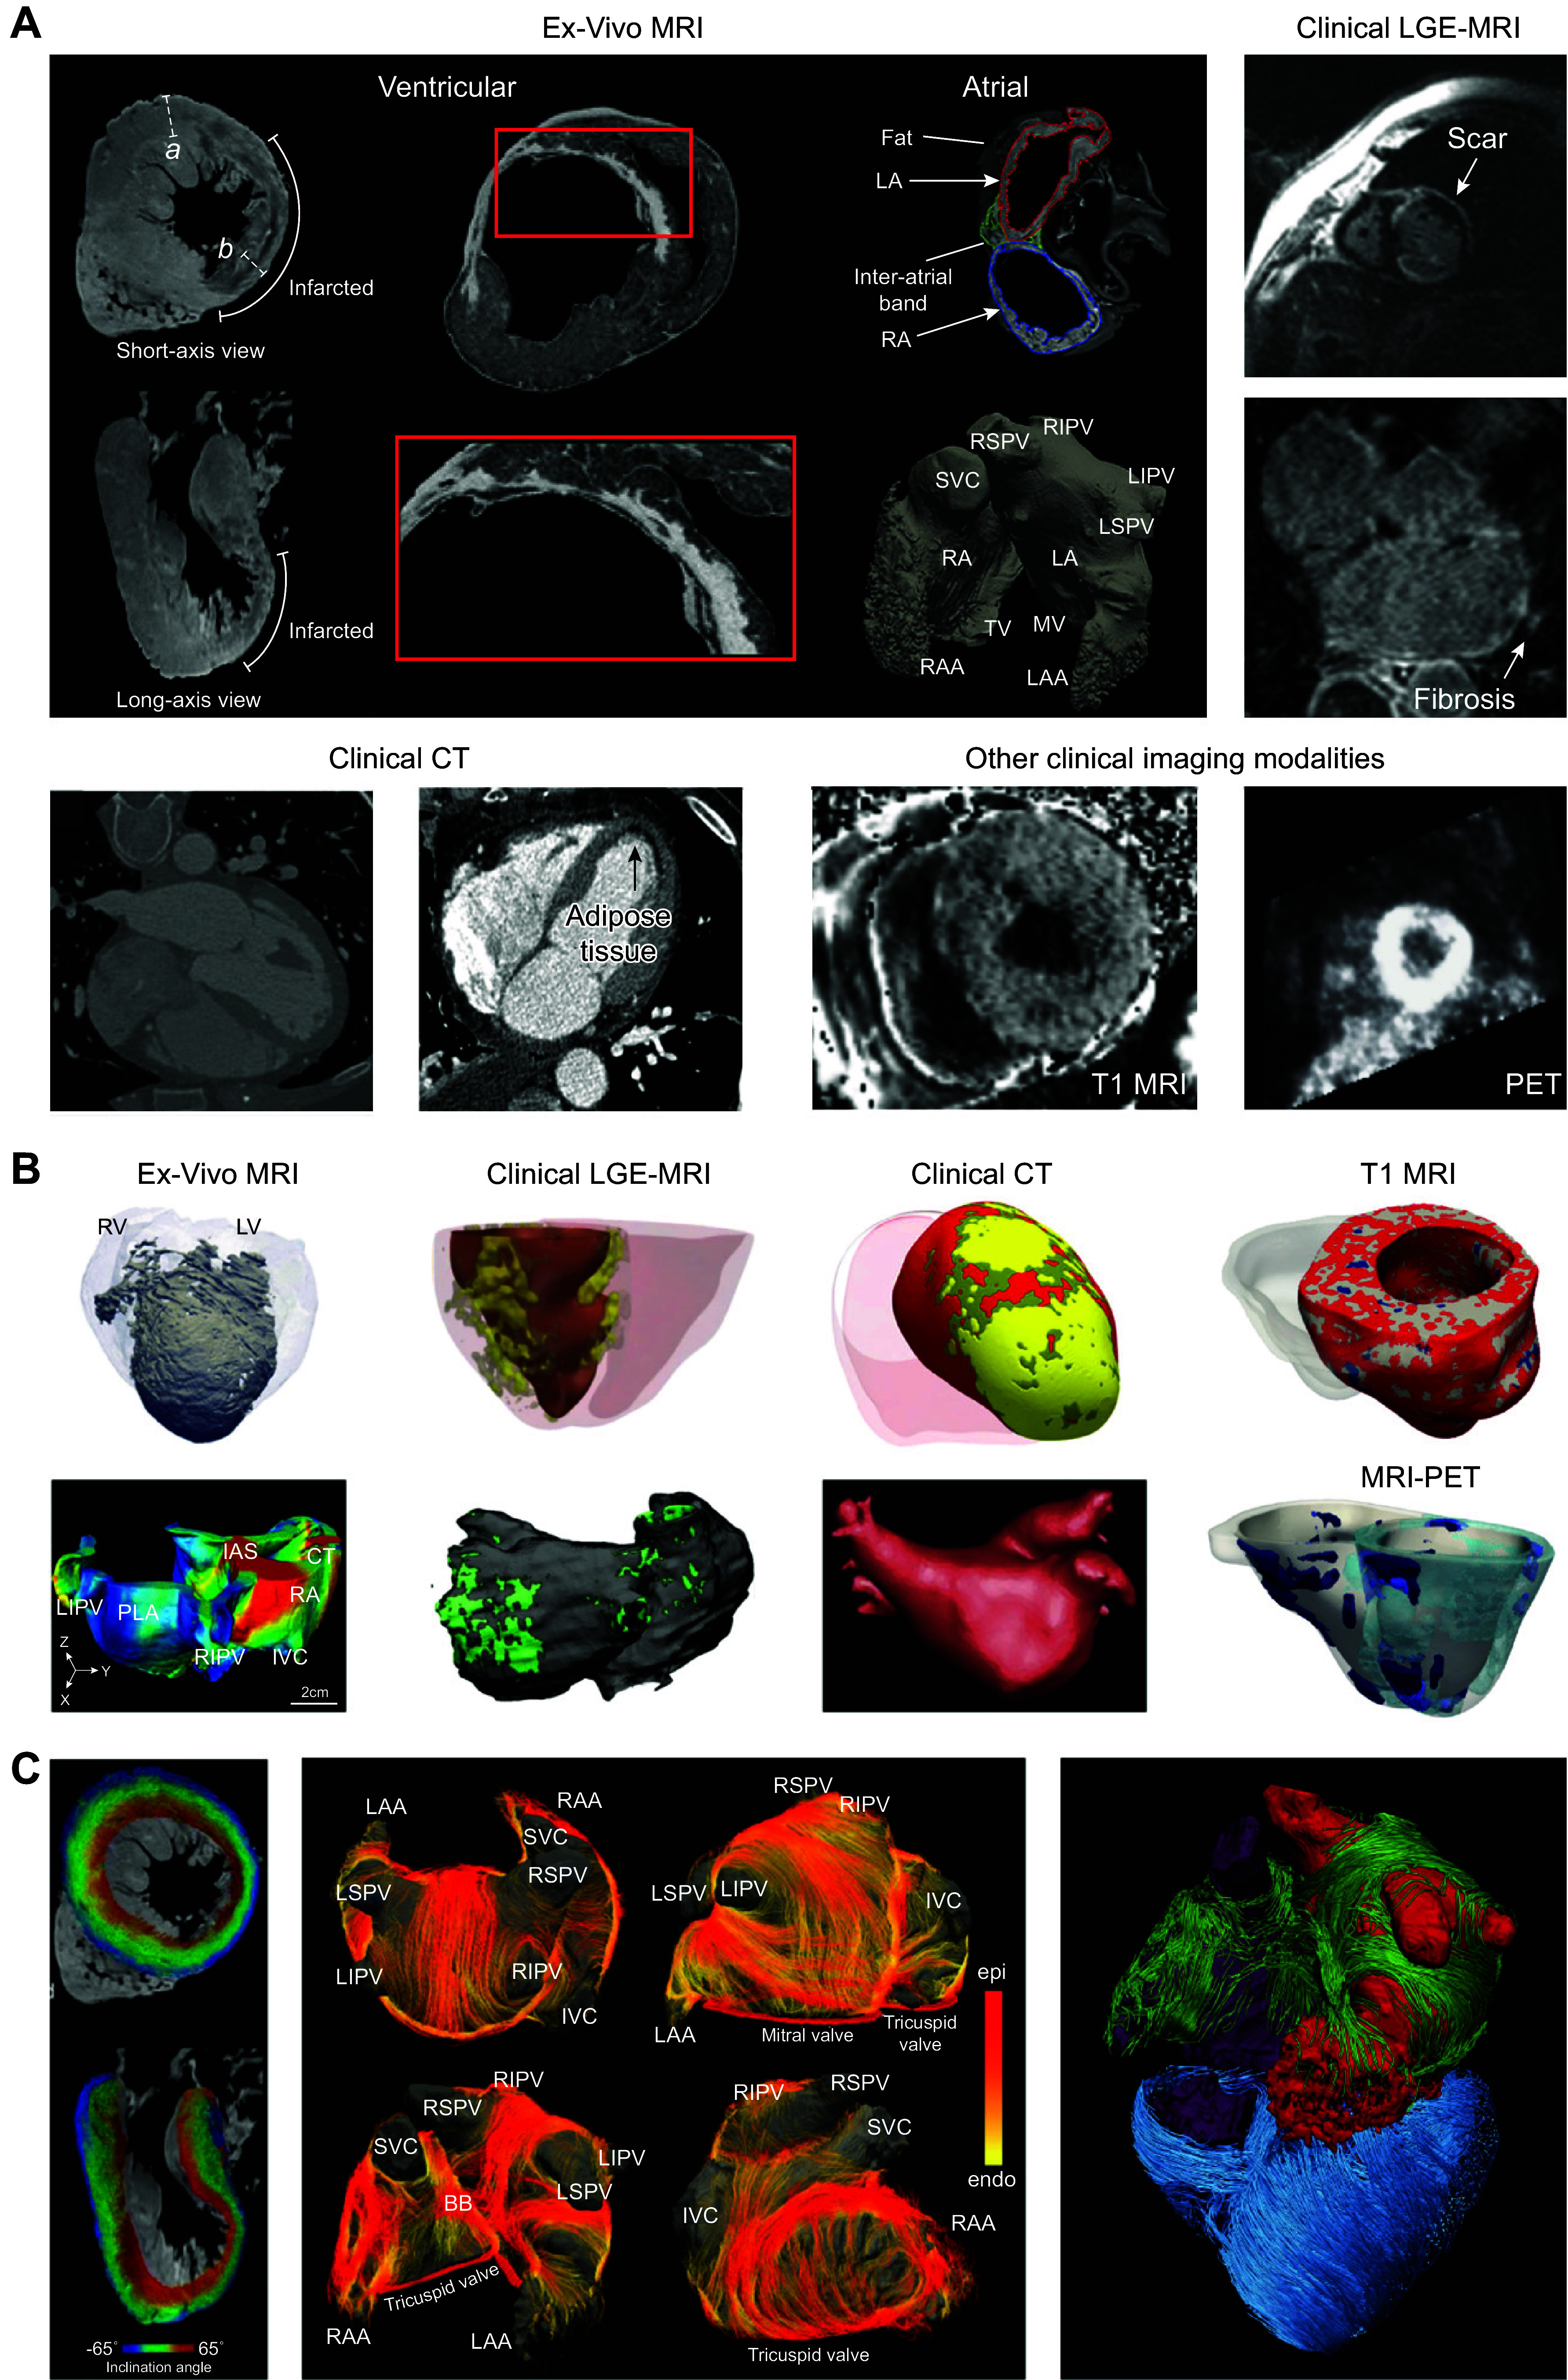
Computational models of the heart. *A*: imaging modalities used in whole heart modeling. Ex vivo images reproduced from Ref. 254, with permission from *Journal of Cardiovascular Magnetic Resonance* (*left*), and Ref. 256 (*center*) and Ref. 255 (*right*), with permission from *Circulation: Arrhythmia and Electrophysiology*. *B*: personalized atrial and ventricular models reconstructed from various imaging modalities. Images of ex vivo ventricular and atrial models reproduced from Ref. 256 (*top*), with permission from *Circulation: Arrhythmia and Electrophysiology*, and Ref. 257 (*bottom*), with permission from *Journal of American Heart Association*. Images of late gadolinium enhancement (LGE)-magnetic resonance imaging (MRI)-based ventricular and atrial models reproduced from Ref. 258 (*top*) and Ref. 259 (*bottom*), with permission from *Nature Biomedical Engineering*. Computed tomography (CT) images reproduced from Ref. 260 (*top*), with permission from *Circulation: Arrhythmia and Electrophysiology*, and Ref. 261 (*bottom*), with permission from *Progress in Biophysics and Molecular Biology*. Image of T1-MRI-based model reproduced from Ref. 262, with permission from *eLife*. Image of MRI-positron emission tomography (PET) model reproduced from Ref. 263, with permission from *Science Advances*. *C*: fiber orientations mapped to personalized heart models. Images reproduced from Ref. 254 (*left*), with permission from *Journal of Cardiovascular Magnetic Resonance*, and Ref. 255 (*center*), with permission from *Circulation: Arrhythmia and Electrophysiology*. BB, Bachmann’s bundle; CT, crista terminalis; IAS, interatrial septum; IVC, inferior vena cava; LA, left atrium; LAA, left atrial appendage; LIPV, left inferior pulmonary vein; LSPV, left superior pulmonary vein; LV, left ventricle; PLA, posterior left atrium; RA, right atrium; RAA, right atrial appendage; RIPV, right inferior pulmonary vein; RSPV, right superior pulmonary vein; RV, right ventricle; SVC, superior vena cava.
